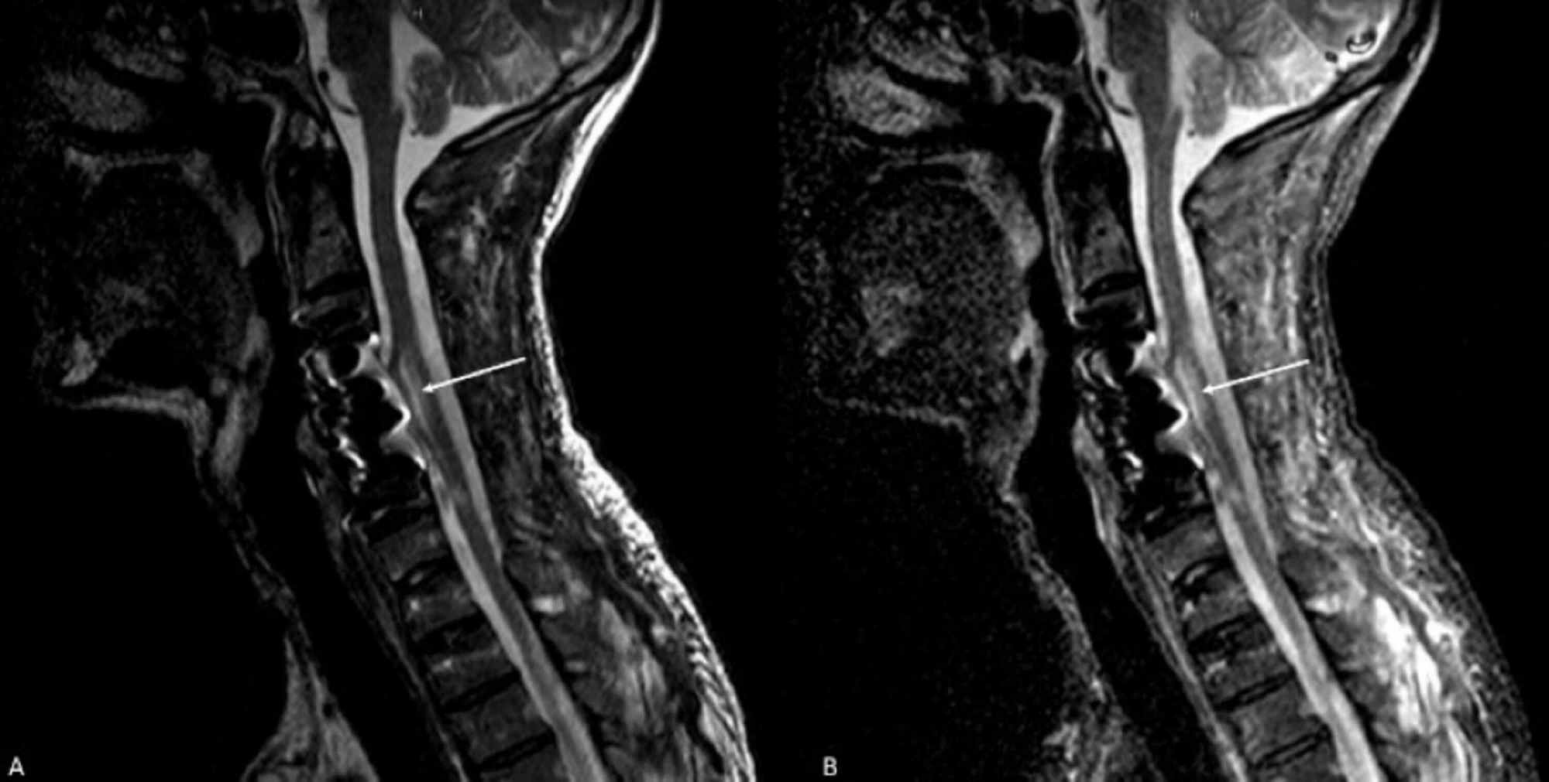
Sagittal MRI of the cervical spine Sagittal MRI of the cervical spine (A) T2 and (B) STIR. The spinal cord shows myelomalacia and cord atrophy at the C4-C7 (arrow). There is also deformity of the spinal cord along the ventral aspect, likely secondary to ventral adhesions of the cord with the metallic graft at the corpectomy site. STIR - short TI inversion recovery
